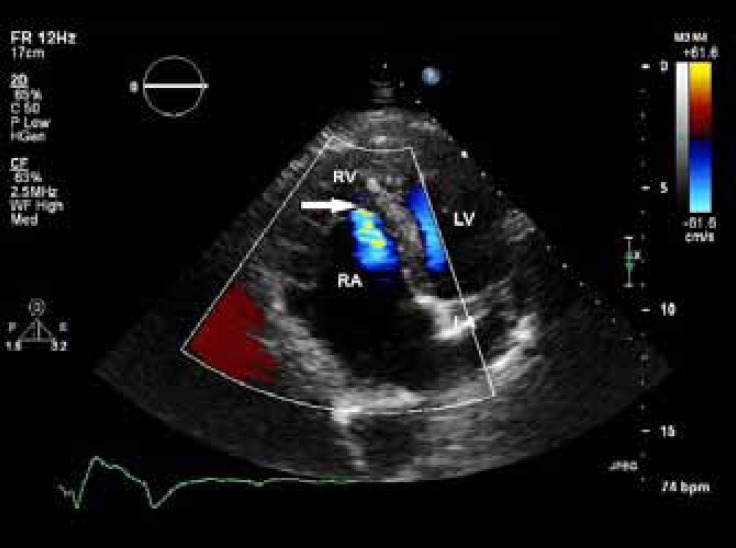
Apical four-chamber view in transthoracic echocardiography, showing Ebstein’s anomaly with mild-to-moderate tricuspid regurgitation (arrow) and right ventricular and atrial dilation
